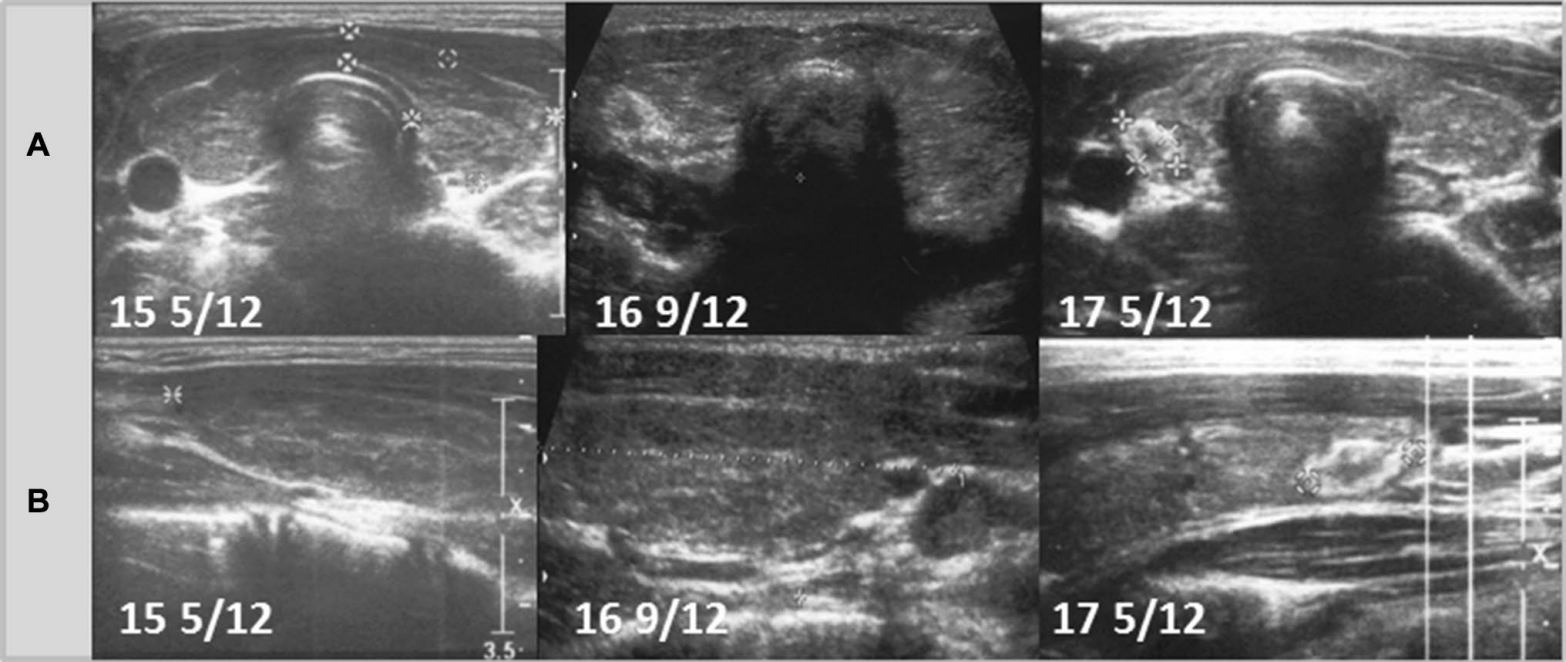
Patient 5. Transversal (a) and longitudinal (b) scans of the hypoechogenic thyroid gland revealed the formation of the nodule 4 11/12 years since referral and 1 4/12 year since last ultrasound assessment. The growth of the nodule was fast from 6.5 × 5.5 × 3.2 mm to 6.6 × 9.8 × 4.1 mm in 8 months (132% increase). The nodule was surrounded by a hyperechogenic border. During follow-up, the echogenicity of the thyroid gland increased
